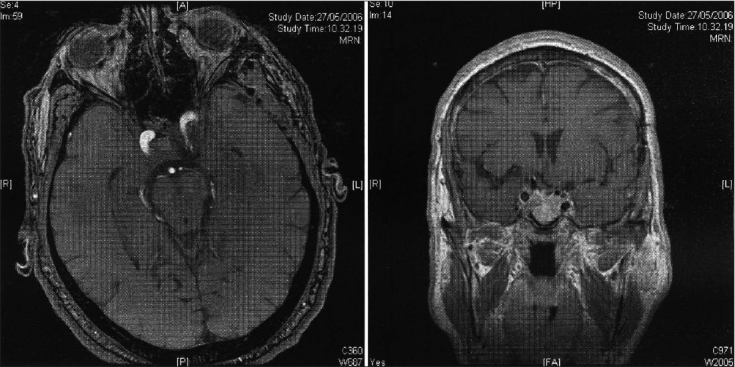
Same patient as in Figures 1, 3, 6. Five-year postoperative axial; a) coronal; Figure b) MRI shows no changes as compared to the earlier postoperative MRI controls
